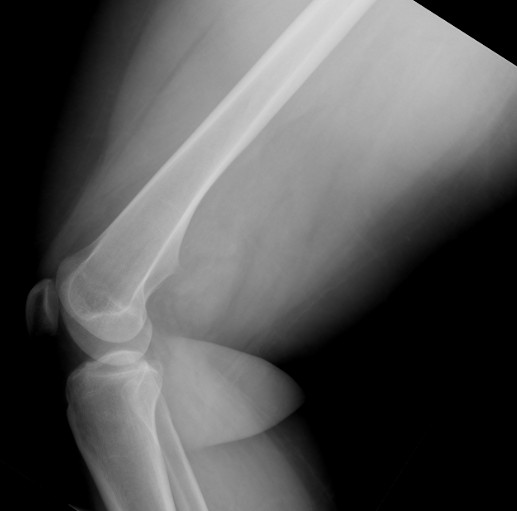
X-ray of the right distal femur (lateral view). The x-ray shows the outline of a broad-based osteochondroma with a sharp tip in a dorsal location.
